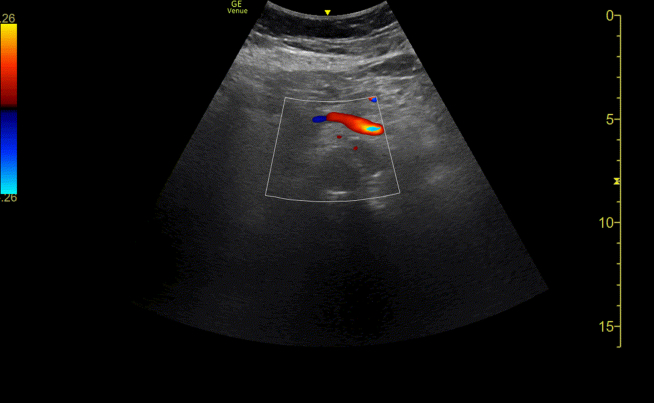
Point-of-care ultrasonography with color Doppler shows a filling defect in the abdominal aorta.
